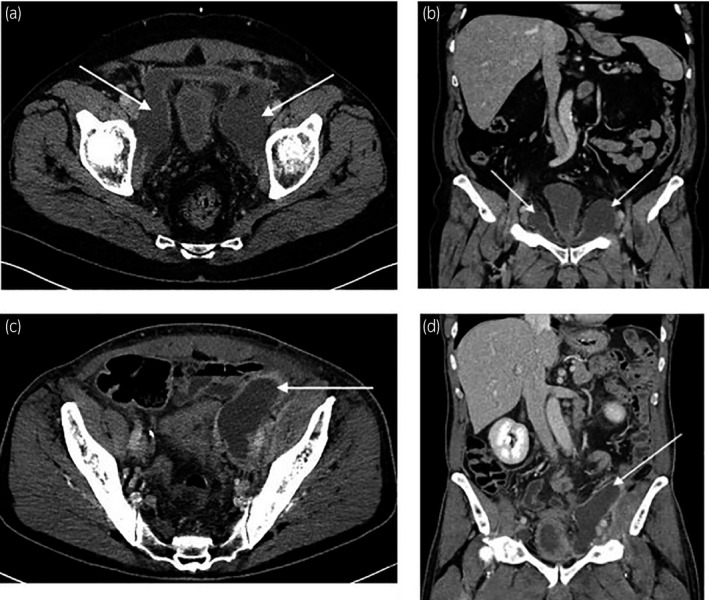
Before lymphatic embolization. (a, b) Patient 1: CT scanner at day 10 after RP, before percutaneous drainage (a, axial slice; b, coronal reformation). PL indicated by the arrow. (c, d) Patient 2: CT scanner showing a voluminous left PL (c, axial slice; d, coronal reformation). Compression of the external iliac vein. PL indicated by the arrow.
